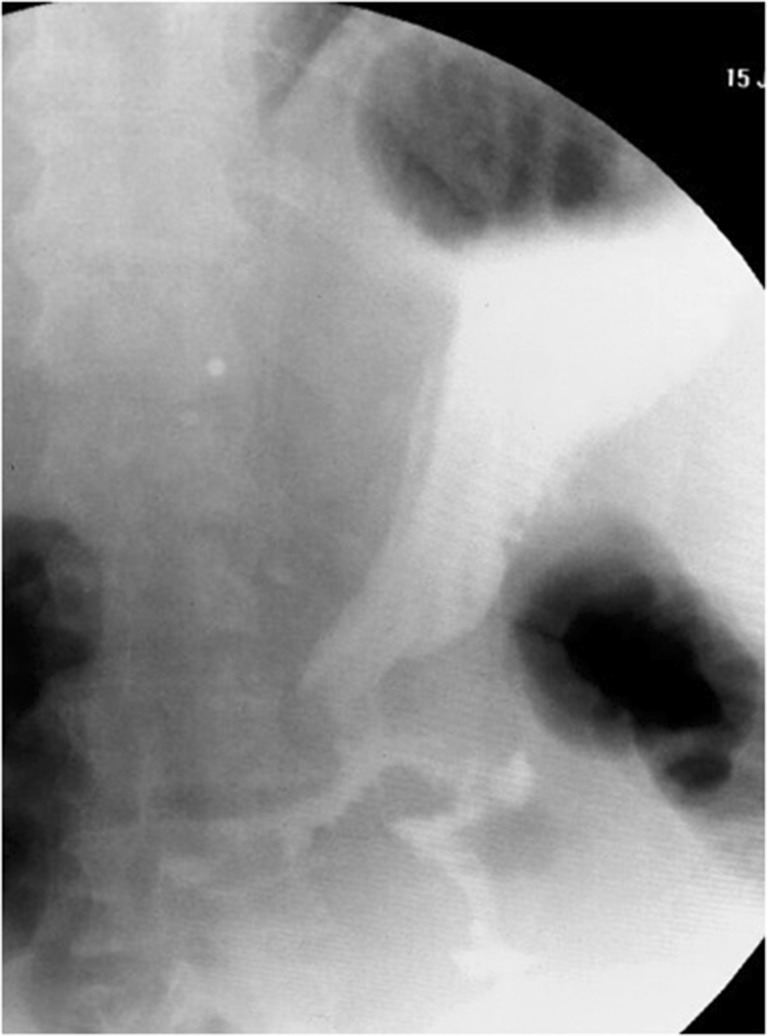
Postoperative X-ray swallow examination shows a preferential way of the contrast through the anastomosis
